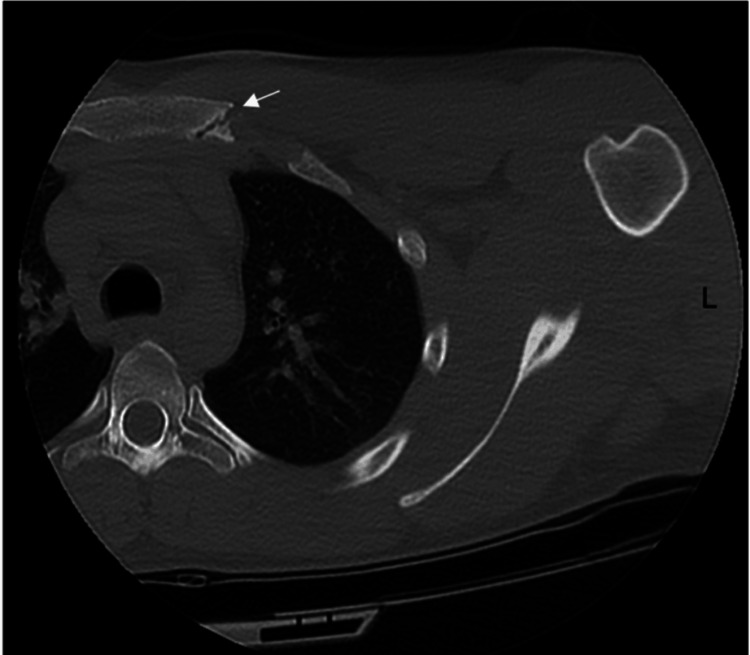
CT scan of the fractured manubrium three months post-injury. Axial view of the chest highlighting non-union of the left posterior aspect of the manubrium with minimal bony healing and sclerosis (white arrow).
